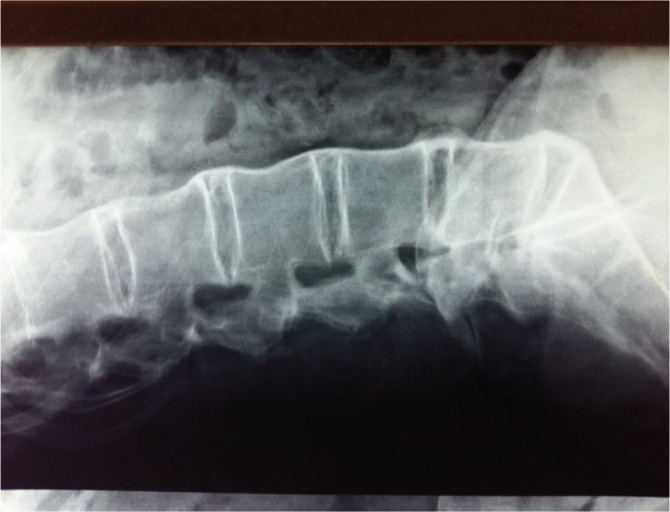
Lumbar spine X-ray with marginal syndesmophytes
